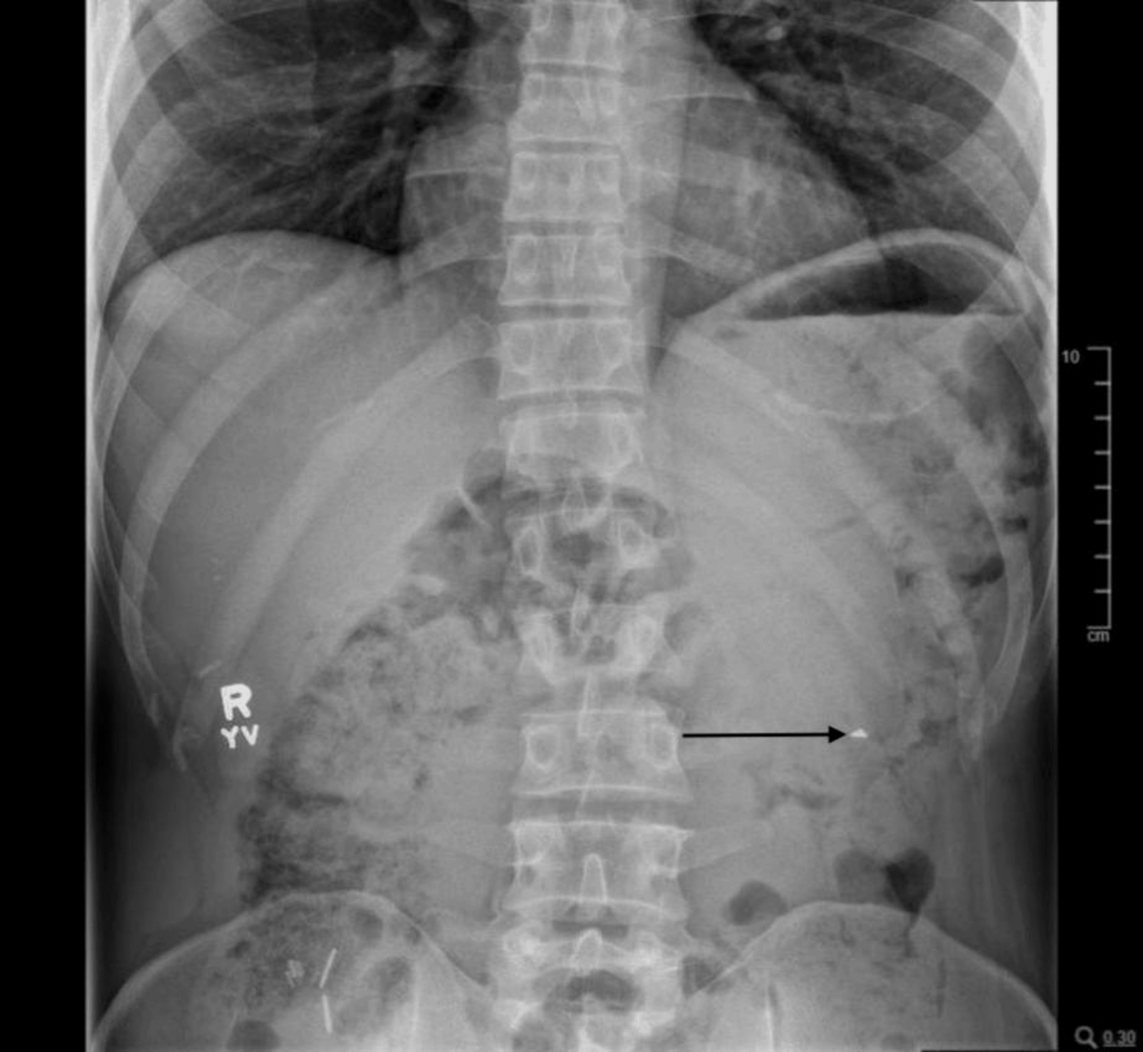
Abdominal radiograph of FB ingestion (see arrow) Abdominal radiograph showing FB in the distal gastric body. FB, foreign body
